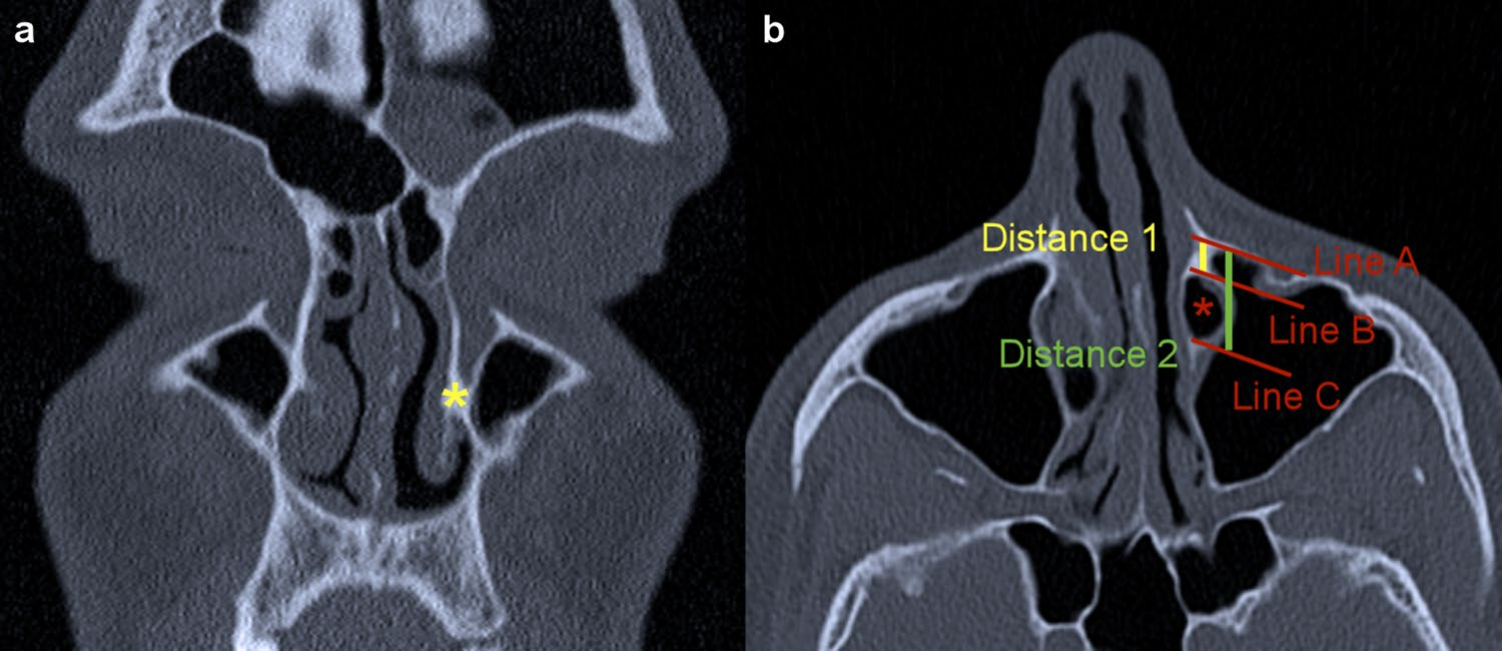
Methodology of measurements. (a) The yellow asterisk marks the anterior insertion point of the inferior turbinate into the frontal process of the maxilla, determined in the coronal CT plane. (b) The yellow line displays the distance between the anterior maxillary sinus wall and the anterior border of the nasolacrimal duct (Distance 1). The difference between distance 1 and distance 2 (green line) represents the width of the nasolacrimal duct (red asterisk). (b) Depicts the corresponding axial CT image to figure a.
